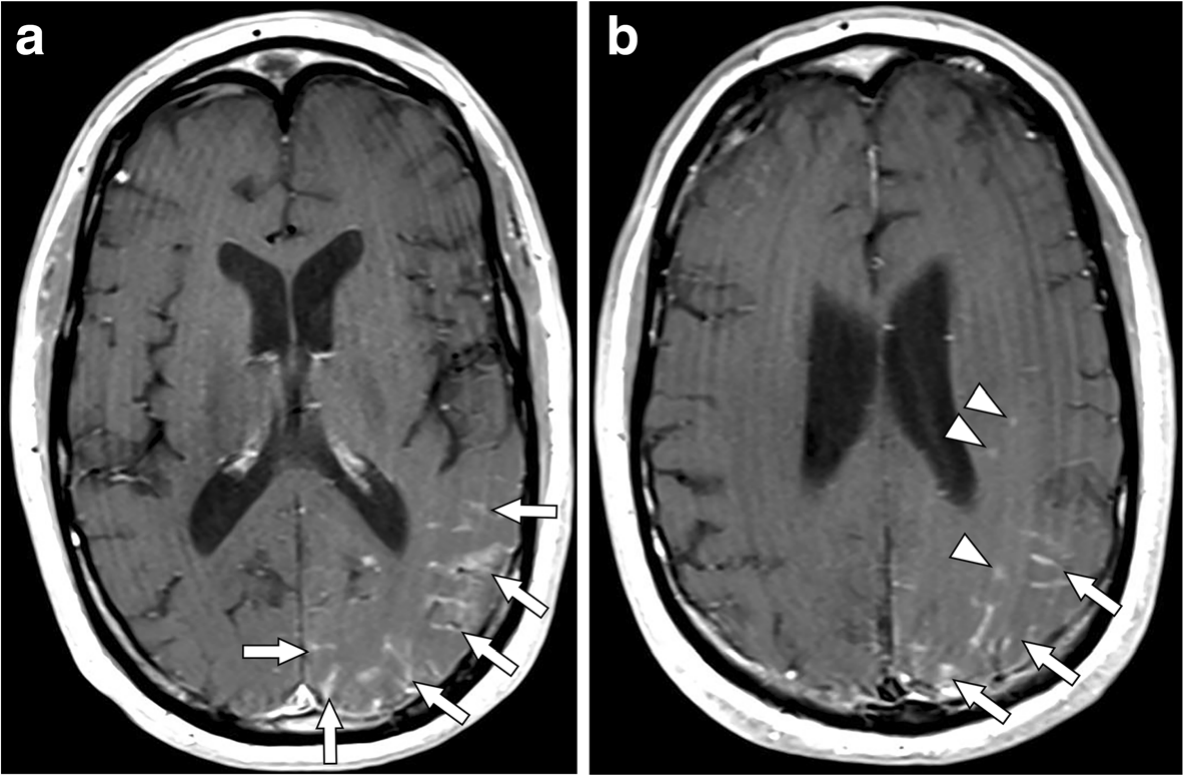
Brain MRI obtained after treatment with steroids in setting of continued neurological complaints. T1-weighted contrast-enhanced axial images at a the level of the internal capsule and b the level of the corona radiata. Note the increased leptomeningeal enhancement (arrows) and new patchy areas of adjacent parenchymal enhancement (arrowheads)
